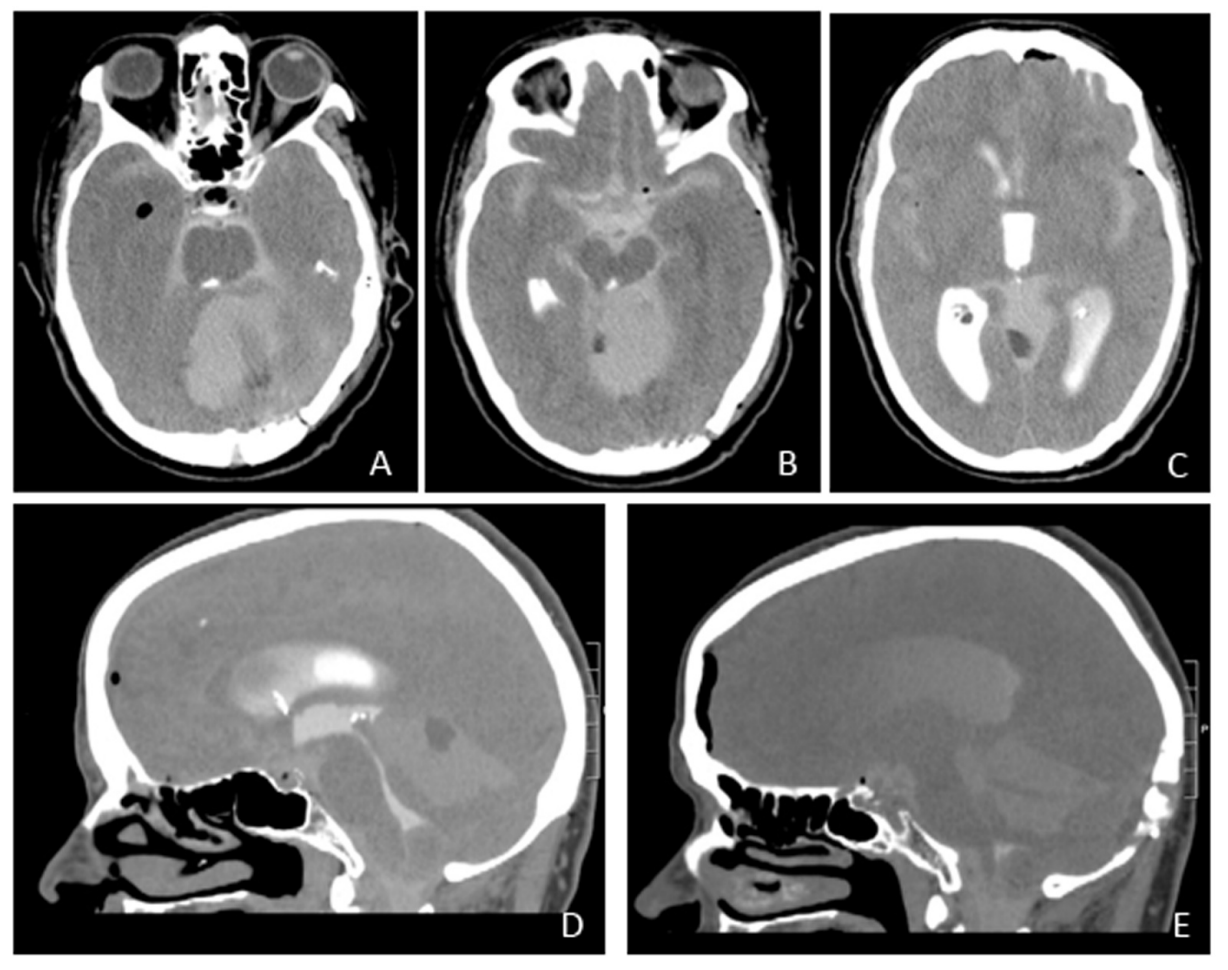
Postoperative CT Cisternogram Images Axial (A-C) and sagittal (D-E) CT cisternogram after surgical fenestration of an arachnoid cyst indicates communication between the CSF of the ventricles and the arachnoid cyst.
